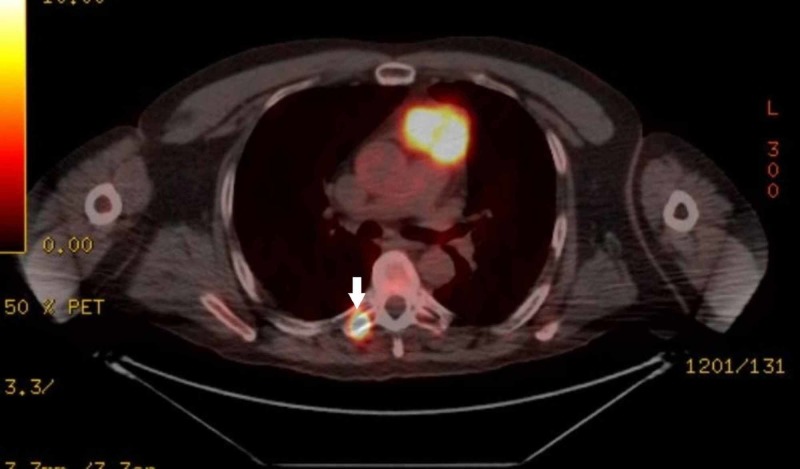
F-fluorodeoxyglucose (FDG) avid lesion within the lateral right T6 transverse process (arrow).
